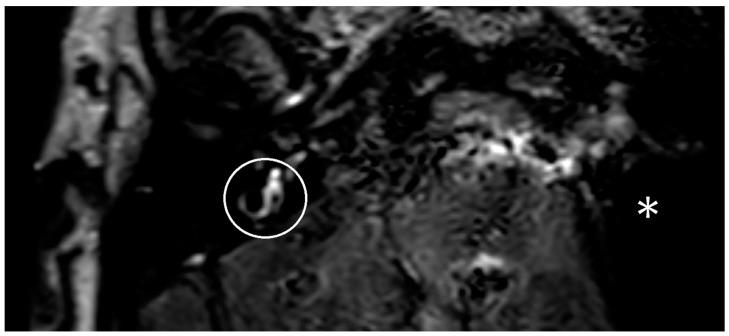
A visualization of a three-dimensional fluid-attenuated inversion recovery (3D-FLAIR) sequence with delayed contrast of the MRI of patient two. The right inner ear is marked with a white circle. Because of an artifact of the implant, the implanted side cannot be seen. The artifact is labeled with a white star. There is no hydrops on the right side.
